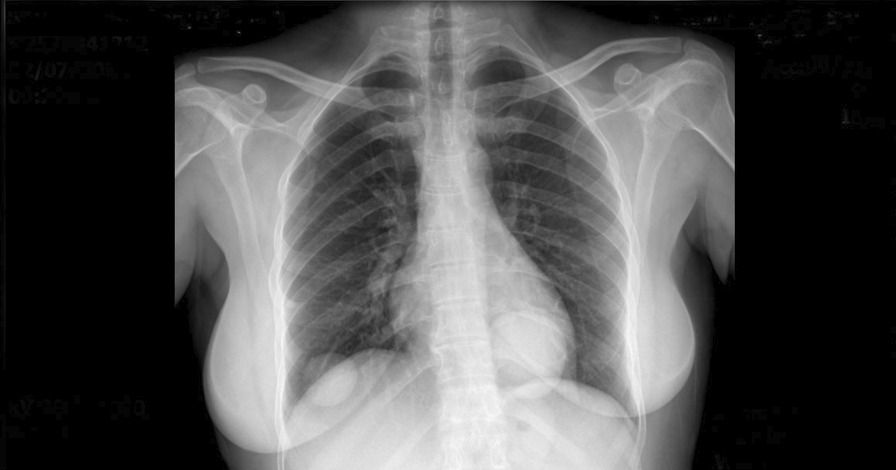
Chest X-ray showing bilateral intraparenchymal lung lesions
